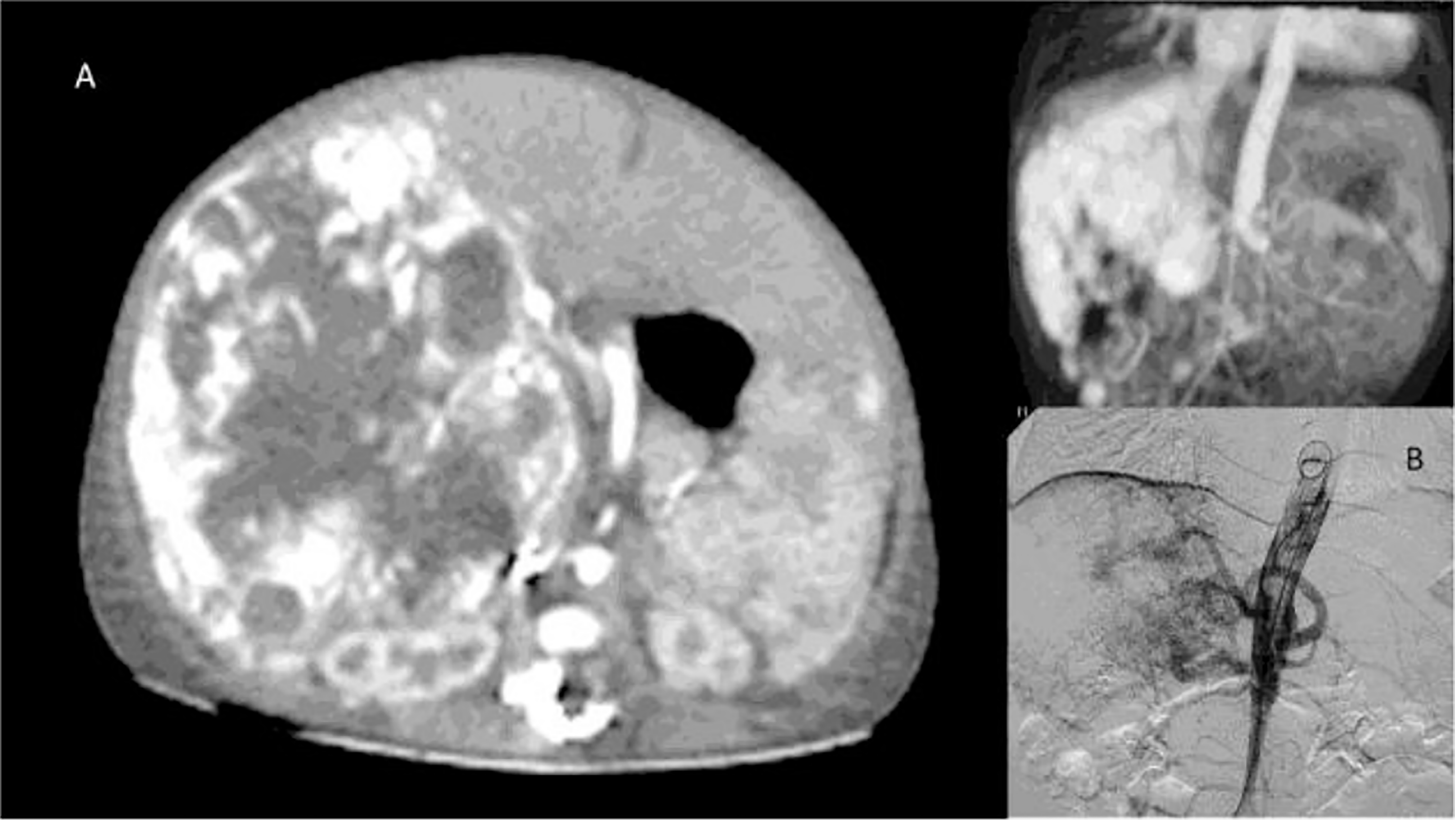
Unifocal hepatic hemangioma (HH) on CT scan (A) and angiography (B).
